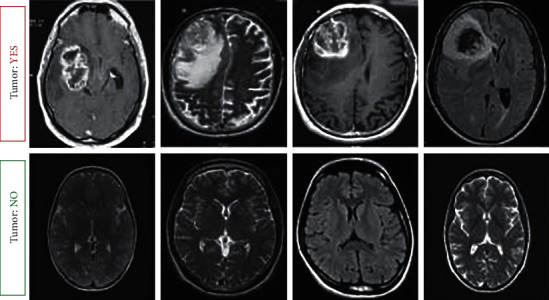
Sample dataset of brain MRI images.
